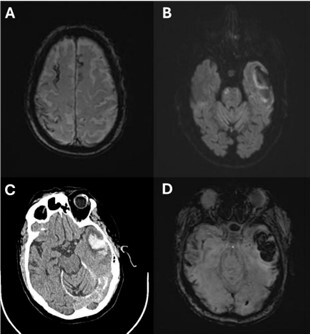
The brain MRI revealed a hypointense image suggestive of superficial siderosis. The brain CT sequence revealed a hyperdense image suggestive of bleeding.
